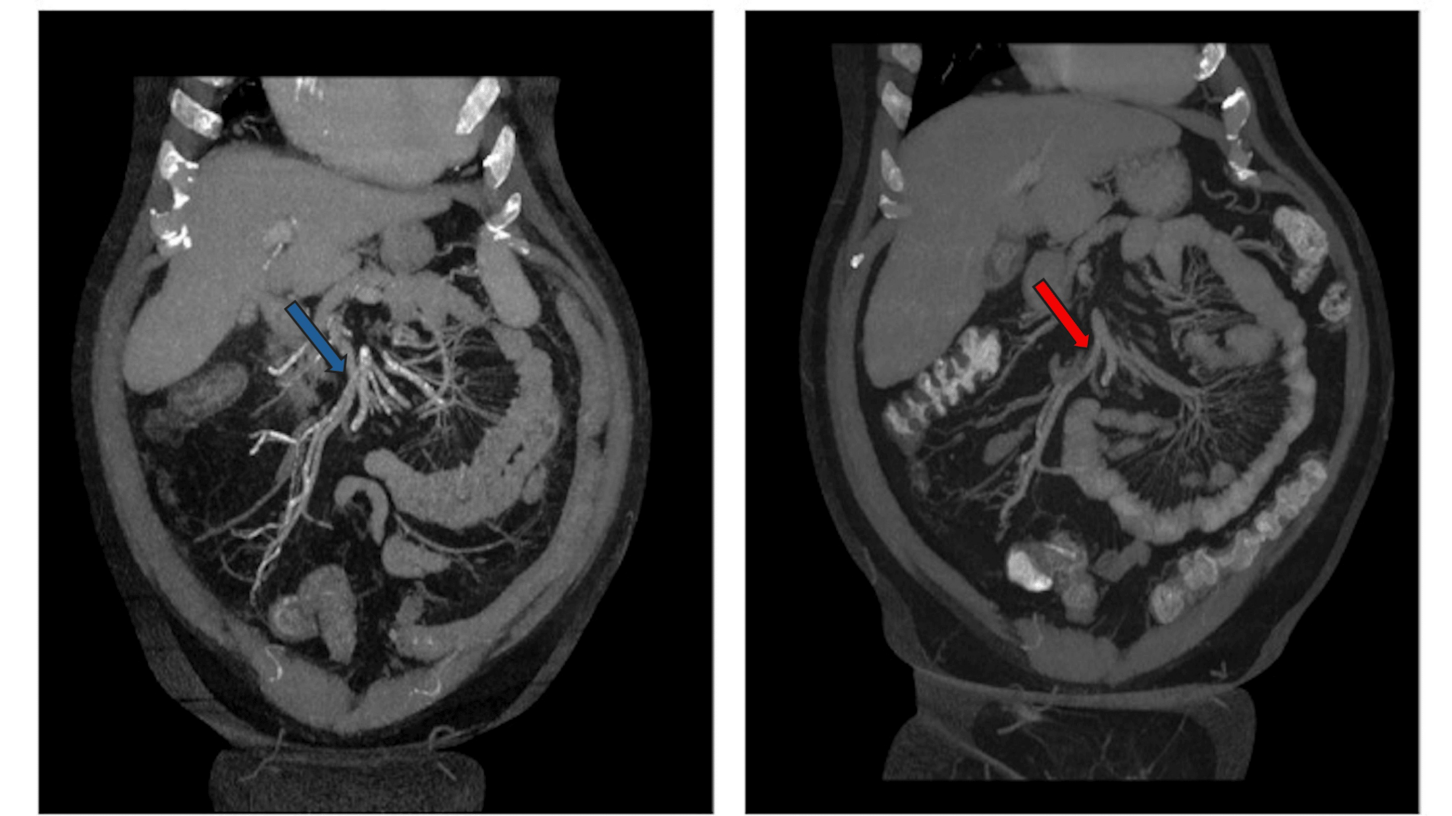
Computed Tomography (CT) abdomen and pelvis following intravenous contrast administration in portal venous phase. Images were obtained with a time difference of 3 years. On baseline examination (Right image), there was moderate diffuse calcified atherosclerosis affecting the abdominal aorta and main abdominal and pelvic branches (red arrow). Follow-up examination (left image) showed significant interval worsening especially involving splanchnic and mesenteric vasculature (blue arrow), bilateral iliac axis and deep pelvic branches.
